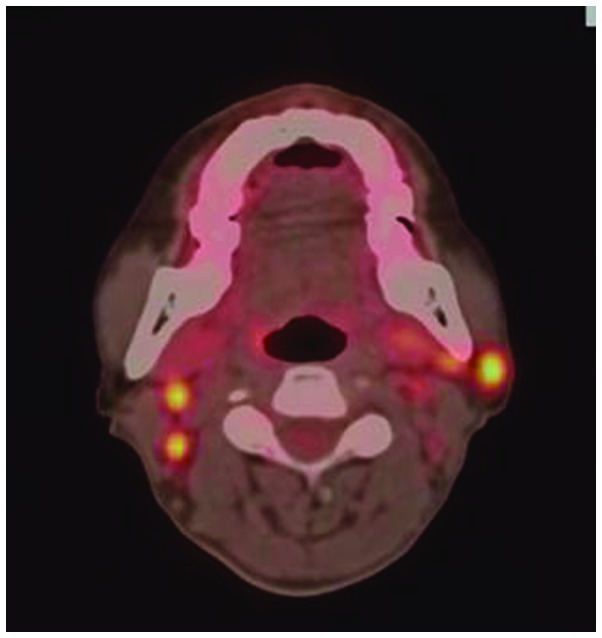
^18^F-fluorodeoxyglucose uptake in cervical lymph nodes as revealed by positron emission tomography/computed tomography.
